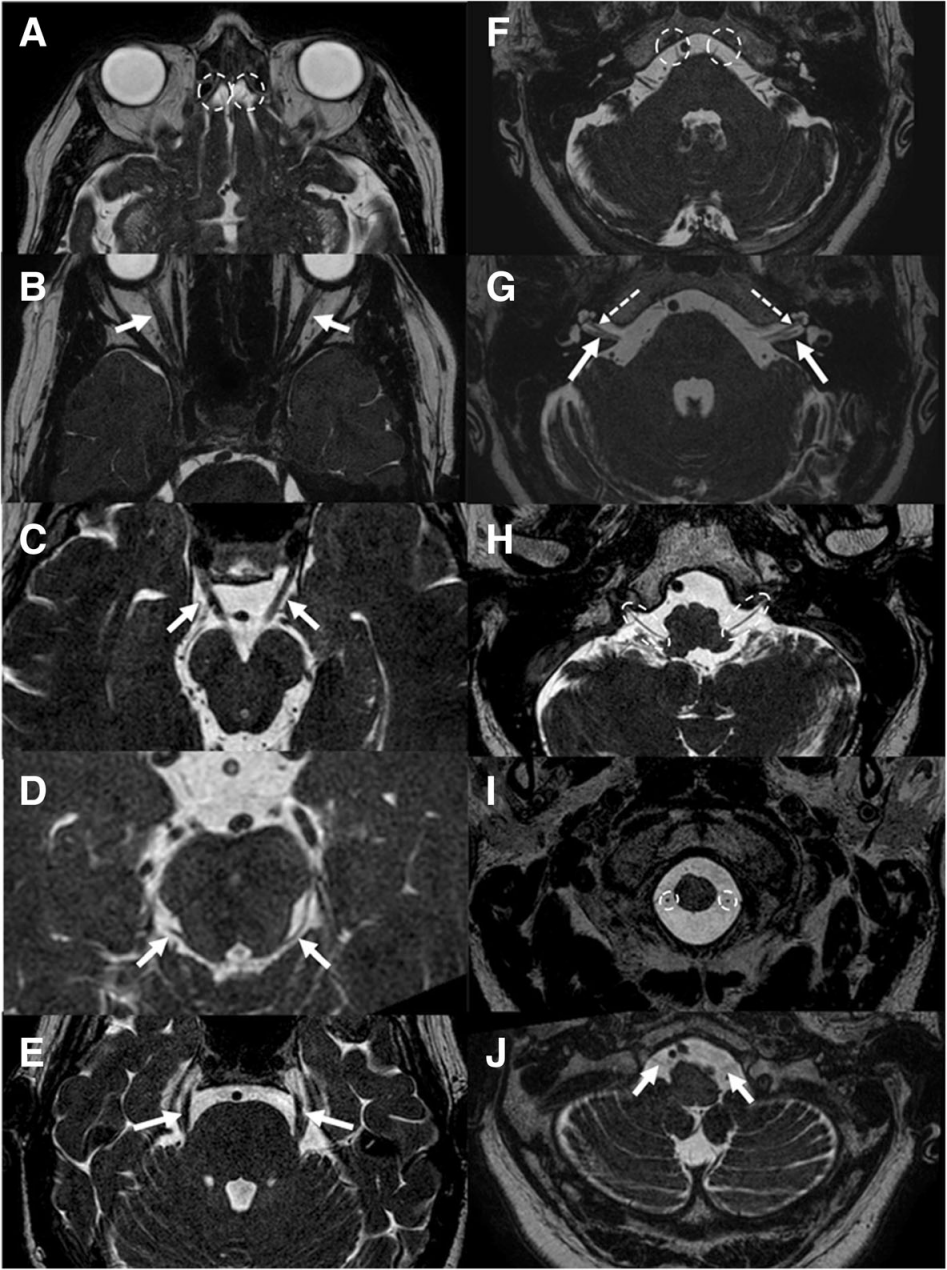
MRI of the cisternal tracts of normal cranial nerves. Steady-state free procession (SSFP), axial planes. a Olfactory (circles); b optic (arrows); c oculomotor (arrows); d trochlear (arrows); e trigeminal (arrows); f abducens (circles); g facial (thin and dotted arrows) and vestibulocochlear (thick arrows); h glossopharyngeal and vagus nerve (circles); i spinal accessory (circles); j hypoglossal (arrows)
